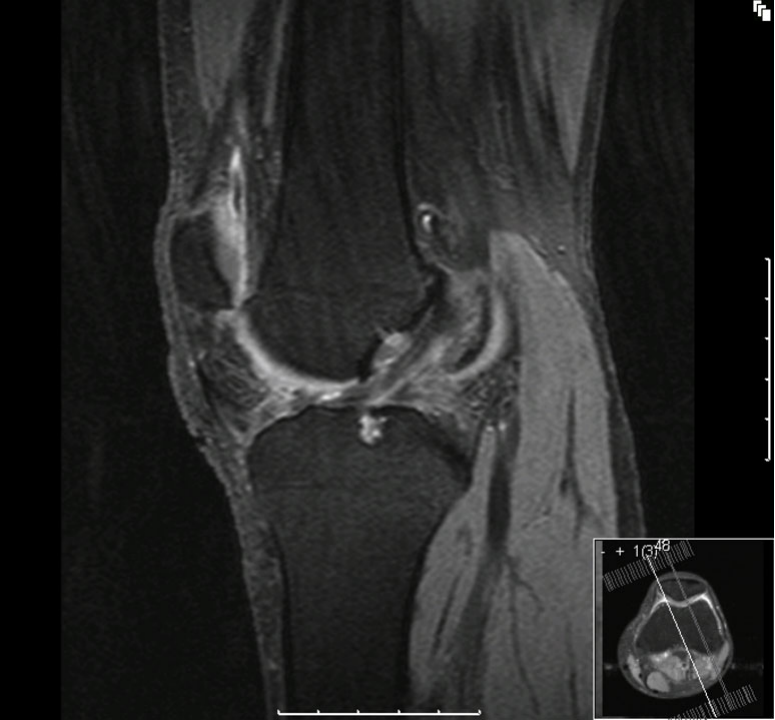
T2 weighted MRI image demonstrating intact anterior cruciate ligament.
